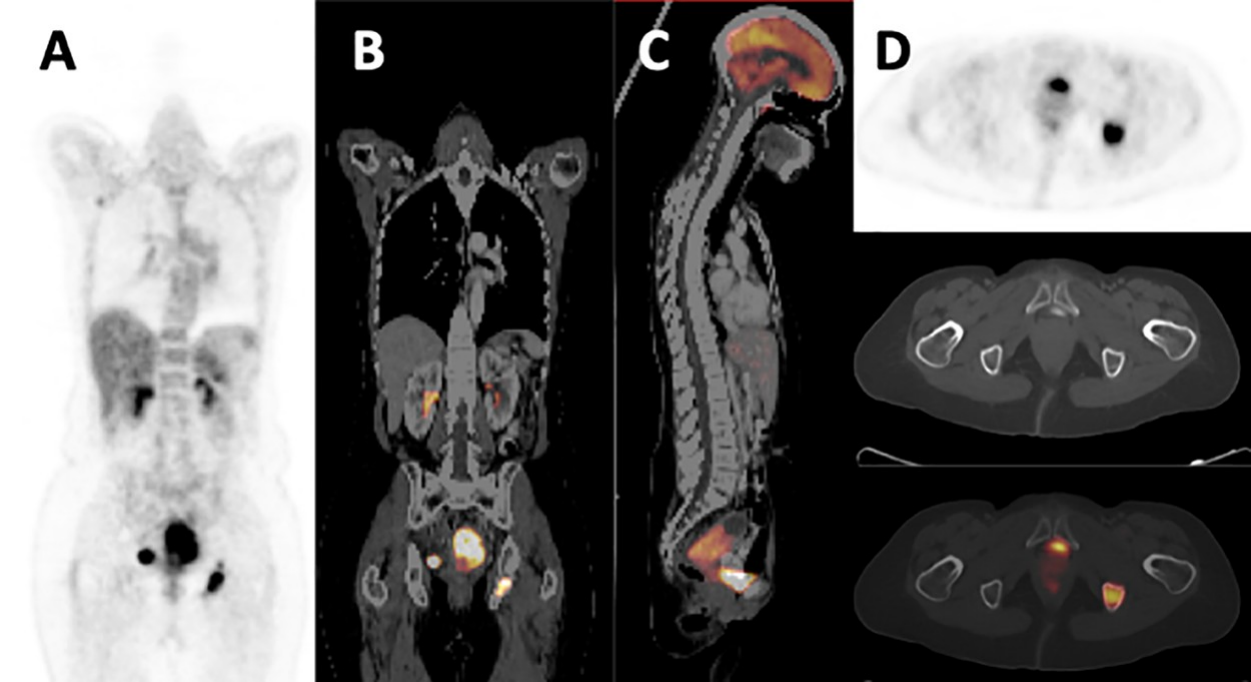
FDG-PET/CT images of a 51-year-old HIV-uninfected female refereed with stage IIIB squamous cell carcinoma of the cervix. (A) Coronal PET, (B) coronal fused PET/CT, (C) sagittal fused PET/CT and (D) axial PET, CT and fused PET/CT images show local disease in the cervix (SUVmax = 24.7, SUVmean = 5.46, MTV = 182.01, and TLG = 993.77) with distant metastasis to right-sided pelvic lymph node and the left ischial bone. She was upstaged to stage IVB disease on account of ischial bone metastasis.
